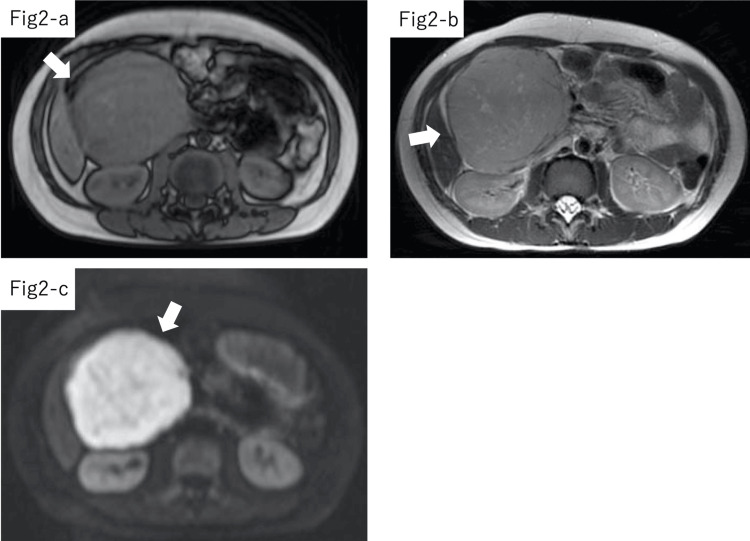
Preoperative MRI findings in Case 3. MRI confirmed the presence of a well-demarcated mass with a solid component of the same intensity as muscle on T1-weighted images (2-A, white arrow), slightly high intensity on T2-weighted images (2-B, white arrow), and extremely high intensity with homogenous enhancement on diffusion-weighted images (2-C, white arrow) in Case 3. MRI: magnetic resonance image
